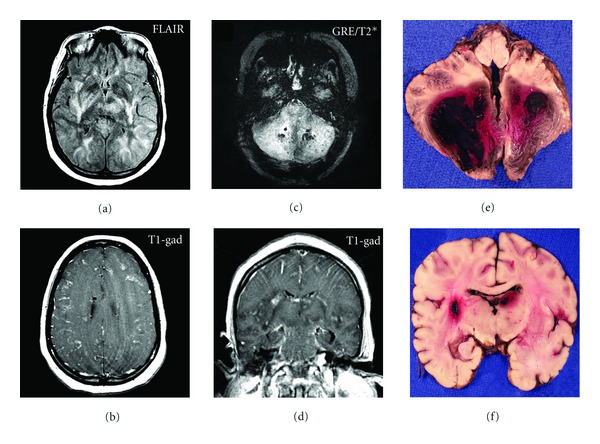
(a) FLAIR MRI sequence demonstrates widespread abnormal signal in the subcortical white matter, internal capsule, putamen, globus pallidus, and thalamus bilaterally. (b) T1 post-gadolinium axial MRI sequence demonstrates diffuse leptomeningeal enhancement over the convexities bilaterally and foci of parenchymal enhancement within the cortex bilaterally. (c) Gradient echo (GRE/T2*) MRI sequence demonstrates hemorrhage in the deep cerebellar white matter bilaterally (mottled black signal, representing magnetic susceptibility). (d) T1 post-gadolinium coronal MRI sequence demonstrates basal leptomeningeal enhancement surrounding the brainstem. (e) Postmortem axial section through the brainstem and cerebellum demonstrates severe bilateral hemorrhage and necrosis of the cerebellar hemispheres. (f) Postmortem coronal section demonstrates widespread but patchy areas of hemorrhage and necrosis in the deep gray nuclei bilaterally and the cerebral hemispheres bilaterally.
